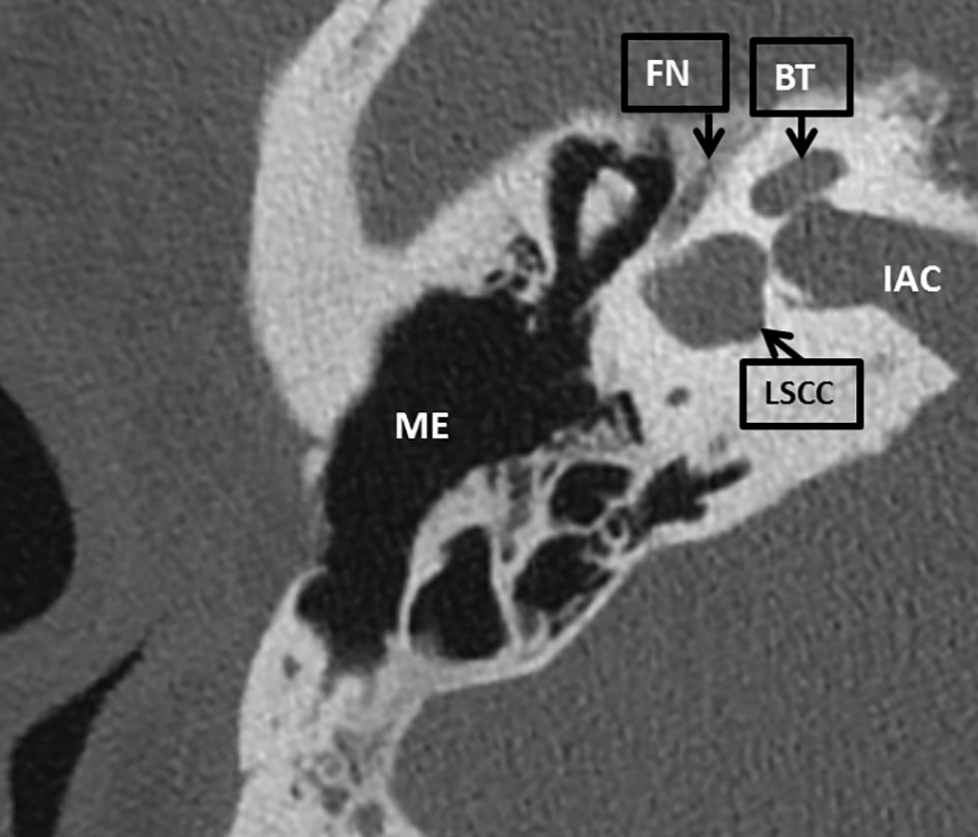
An axial CT scan of the right mastoid bone of patient 1. The lateral semicircular canal is fused with the vestibule to a single cavity. LSCC, lateral semicircular canal; BT, basal turn of the cochlea; IAC, inner auditory canal; FN, facial nerve; ME, middle ear.
